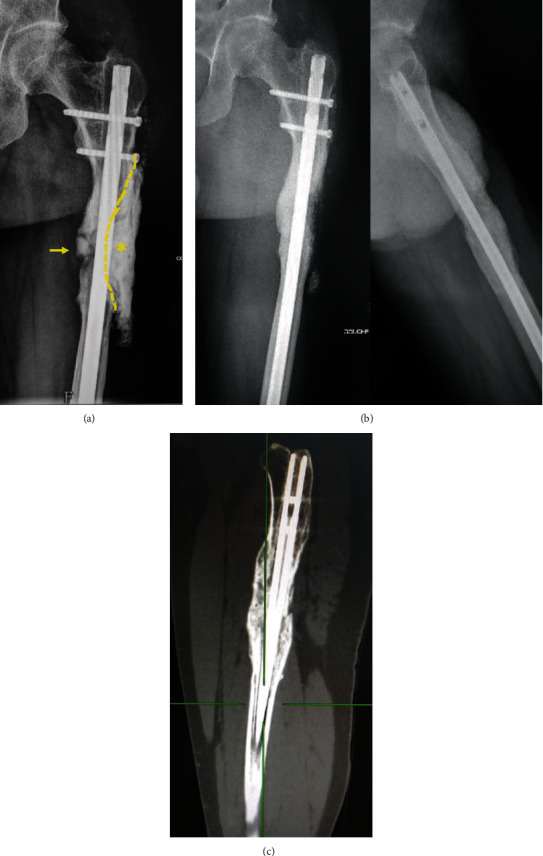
Third procedure. Radiograph showing a fracture of the integrated graft (arrow) and a partial cement spacer (star) into the residual lateral bone defect (a). X-rays (b) and CT-scan (c) demonstrating complete bone union at the last follow-up.
